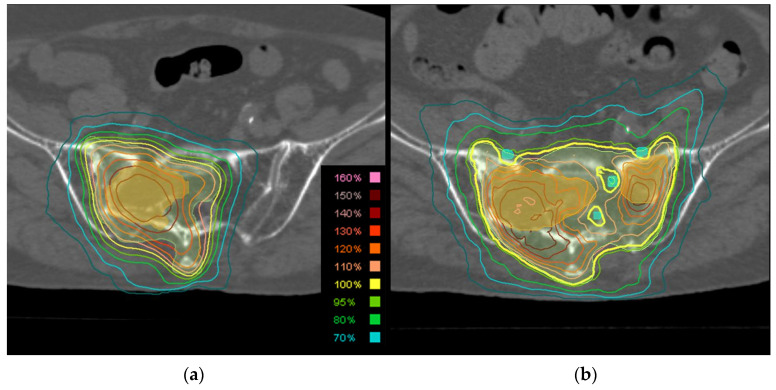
Imaging findings in an 85-year-old male patient with sacral spinal metastases from prostate cancer. (a) Axial computed tomography images with contouring (orange = gross tumor volume and light green = planning target volume) and dose distribution of initial stereotactic body radiotherapy (SBRT) of 24 Gy in two fractions to the S2–5 level. (b) Axial computed tomography images during the second SBRT course, when 30 Gy in five fractions were administered to the S1–5 level. Although the sacral nerves were dose-constrained to <30 Gy, it failed to circumvent the right S1 and S2 nerve roots due to tumor invasion, resulting in almost complete paralysis of the right tibialis anterior muscle after 4 months.
